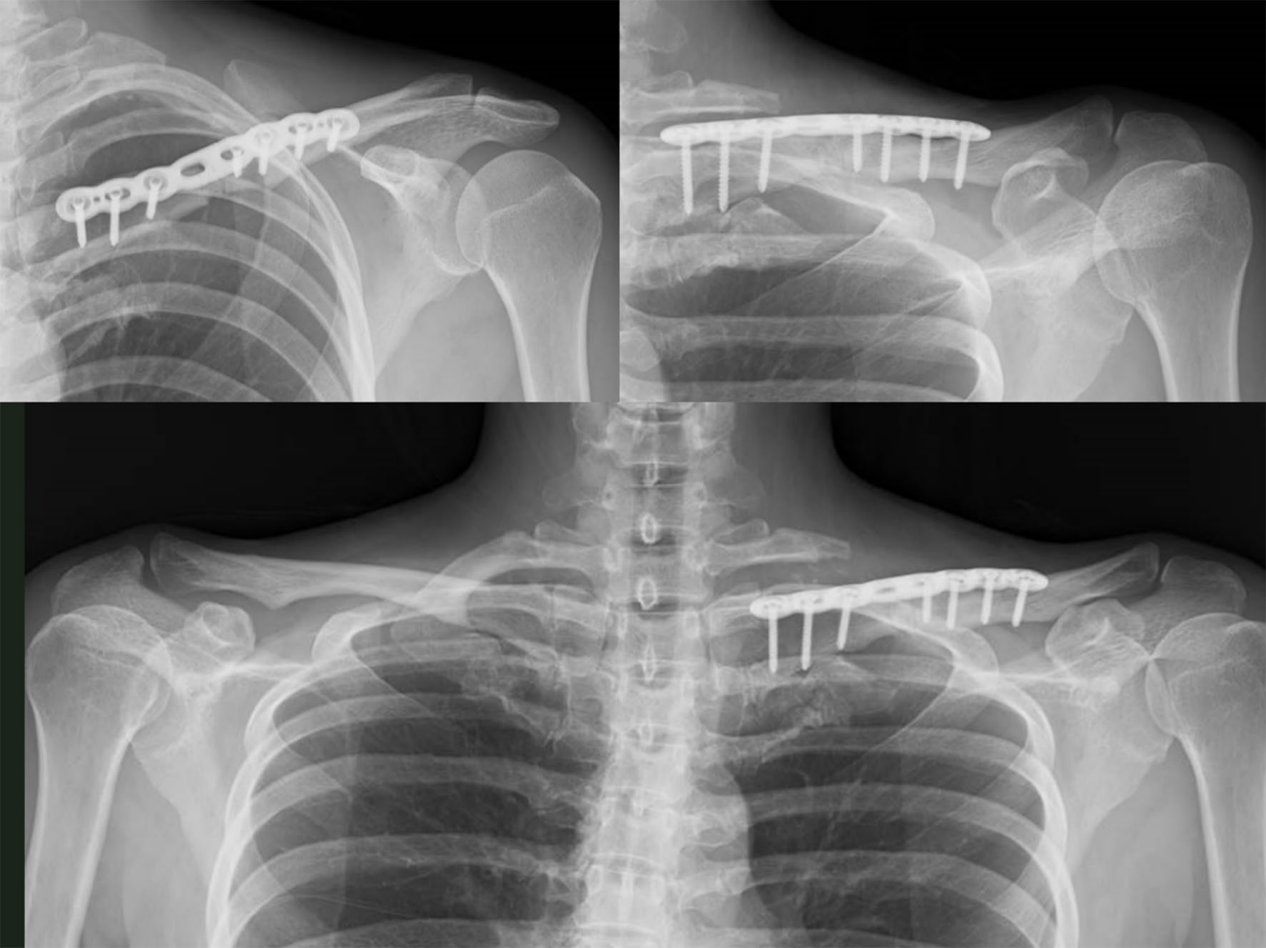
Postoperative radiographs
